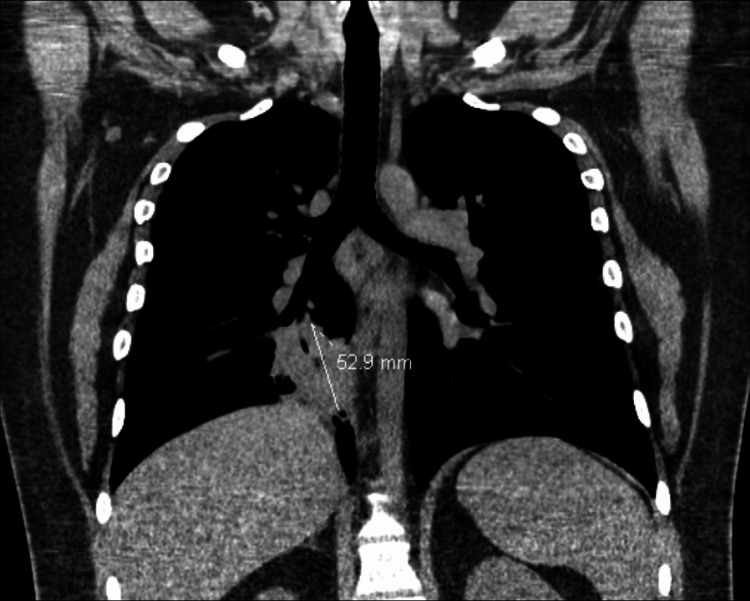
CT chest revealed a moderate-sized focal area of consolidation in the right lung with an intramammary lymph node. A 5.29 cm focal area of consolidation was noted in the right lung. CT: computed tomography
